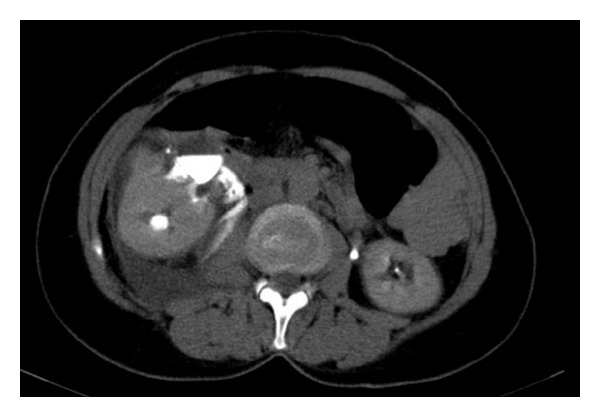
Free passage of contrast material from the pelvis renalis to around the kidney is seen at delayed phase of the contrast enhanced CT scan.
